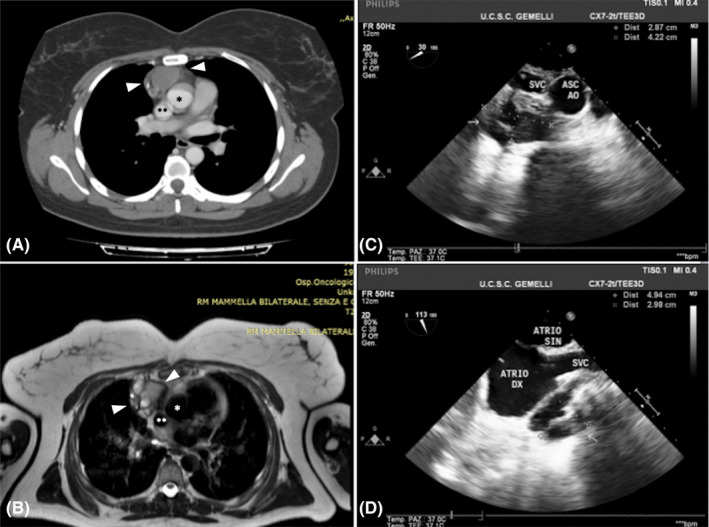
Chest computed tomography scan (A) and magnetic resonance imaging (B) showing the structure and the anatomical relations of the mediastinal mass (white pointers). In particular, the ascending aorta (*) and the superior vena cava () surround the posterior and medial margins of the mass. Transesophageal echocardiogram (C, D) confirms the strict adherence of the mass (arrows) to the superior vena cava (SVC), right atrium (ATRIO DX), and ascending aorta (ASC AO)
